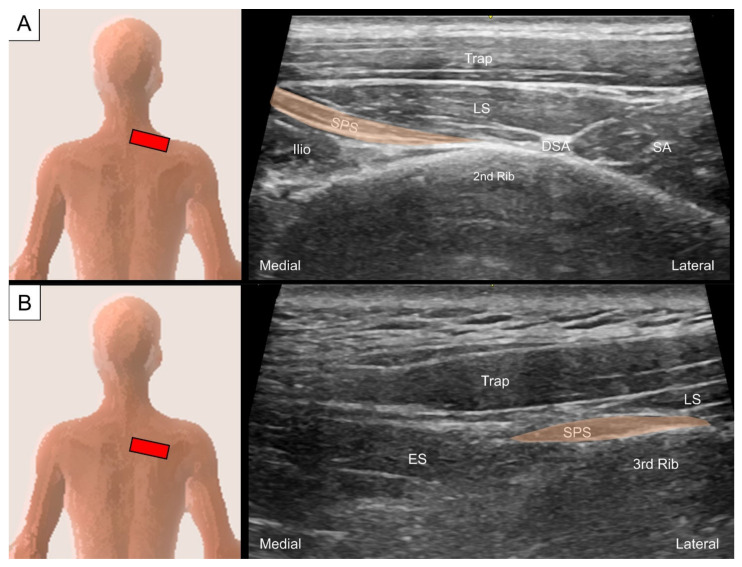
Ultrasound imaging of the serratus posterior superior (SPS) attaching to the (A) second and (B) third ribs. Trap, trapezius; LS, levator scapulae; SA, serratus anterior; DSA, dorsal scapular artery; ilio, iliocostalis; ES, erector spinae.
